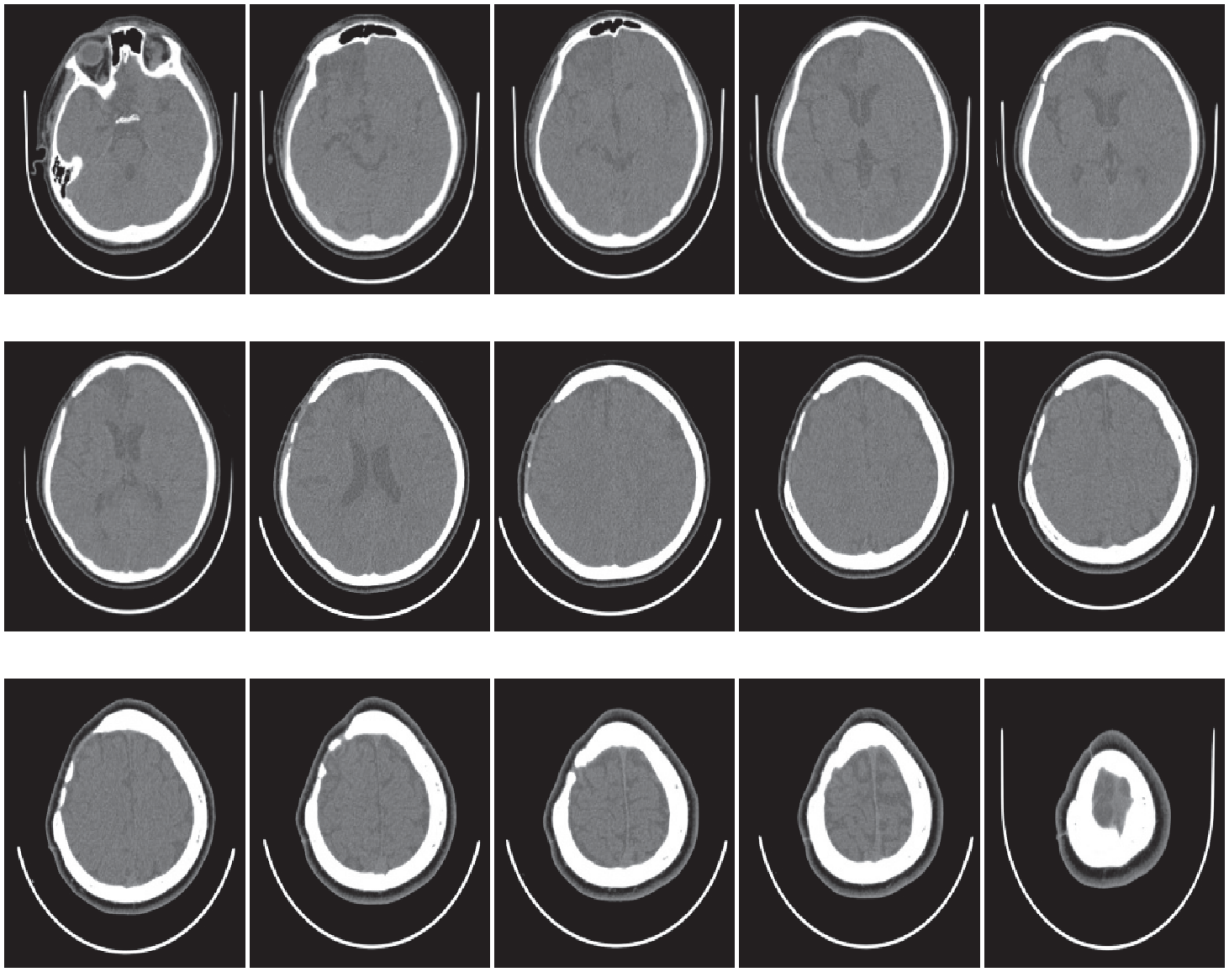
Given CT scanned images of patient with parietal bone fracture.
